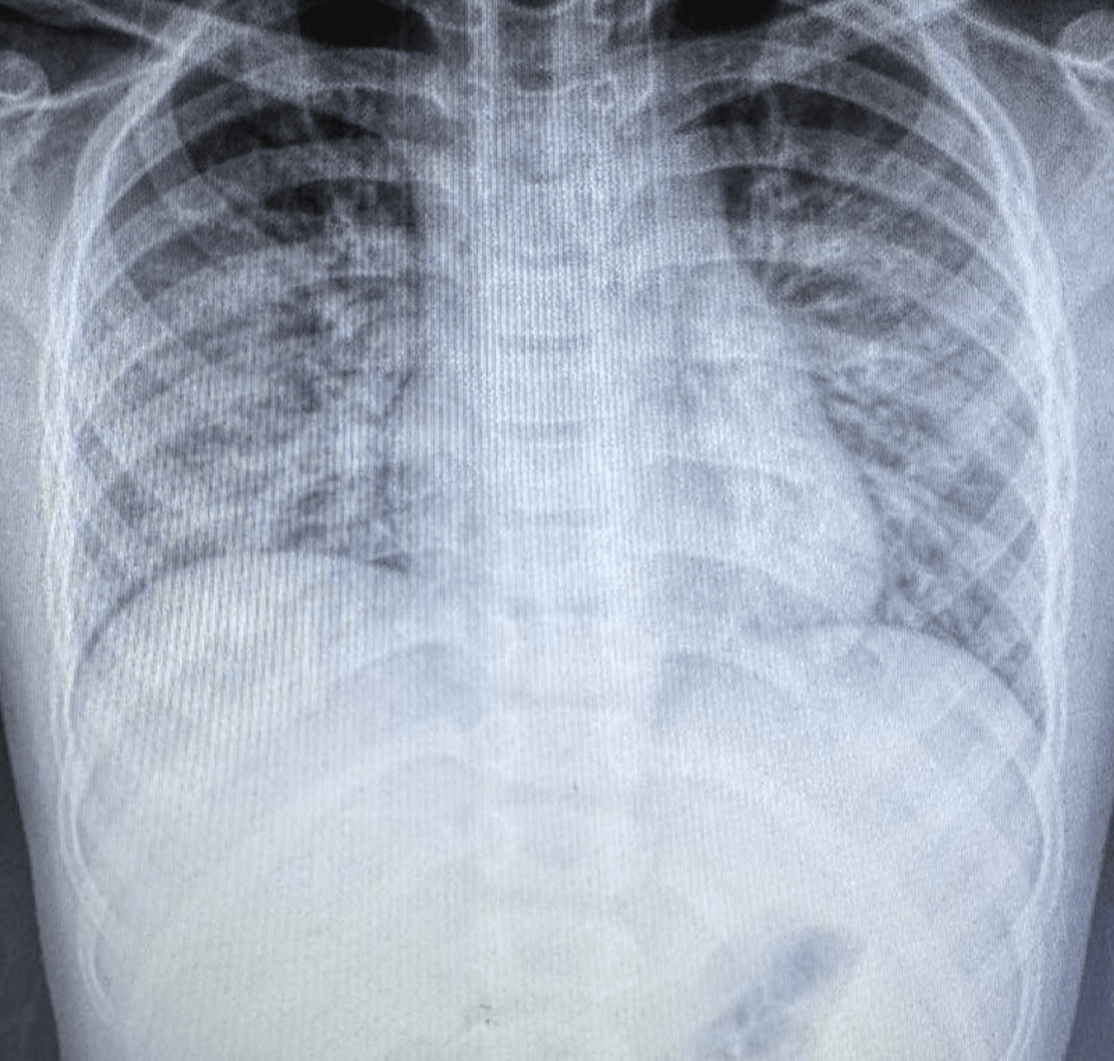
Chest X-ray PA view showing diffuse bilateral opacities PA: posteroanterior
